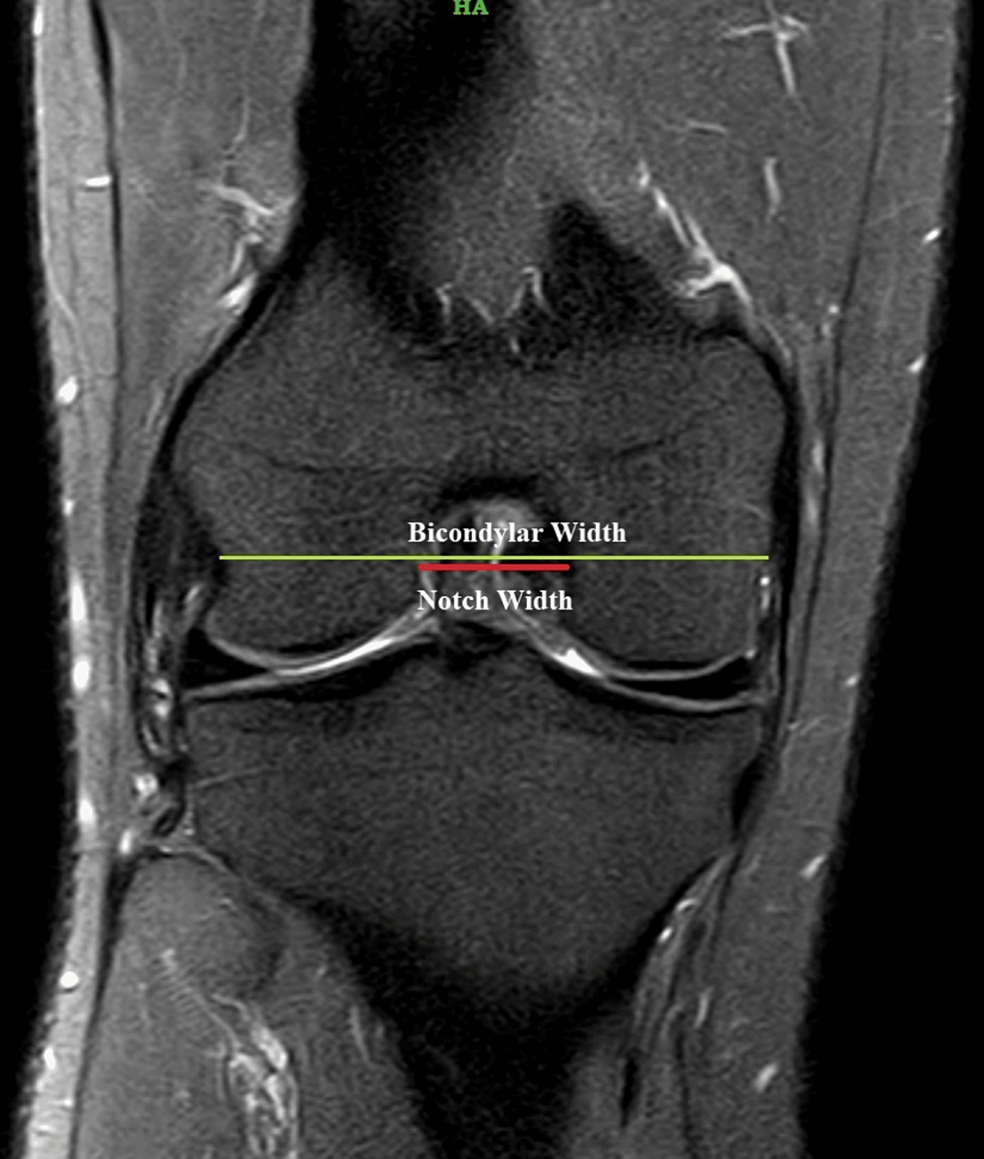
Coronal MRI image: proton density (PD) weighted fast spin echo (FSE) depicting the bicondylar width (green line) and notch width (red line)
